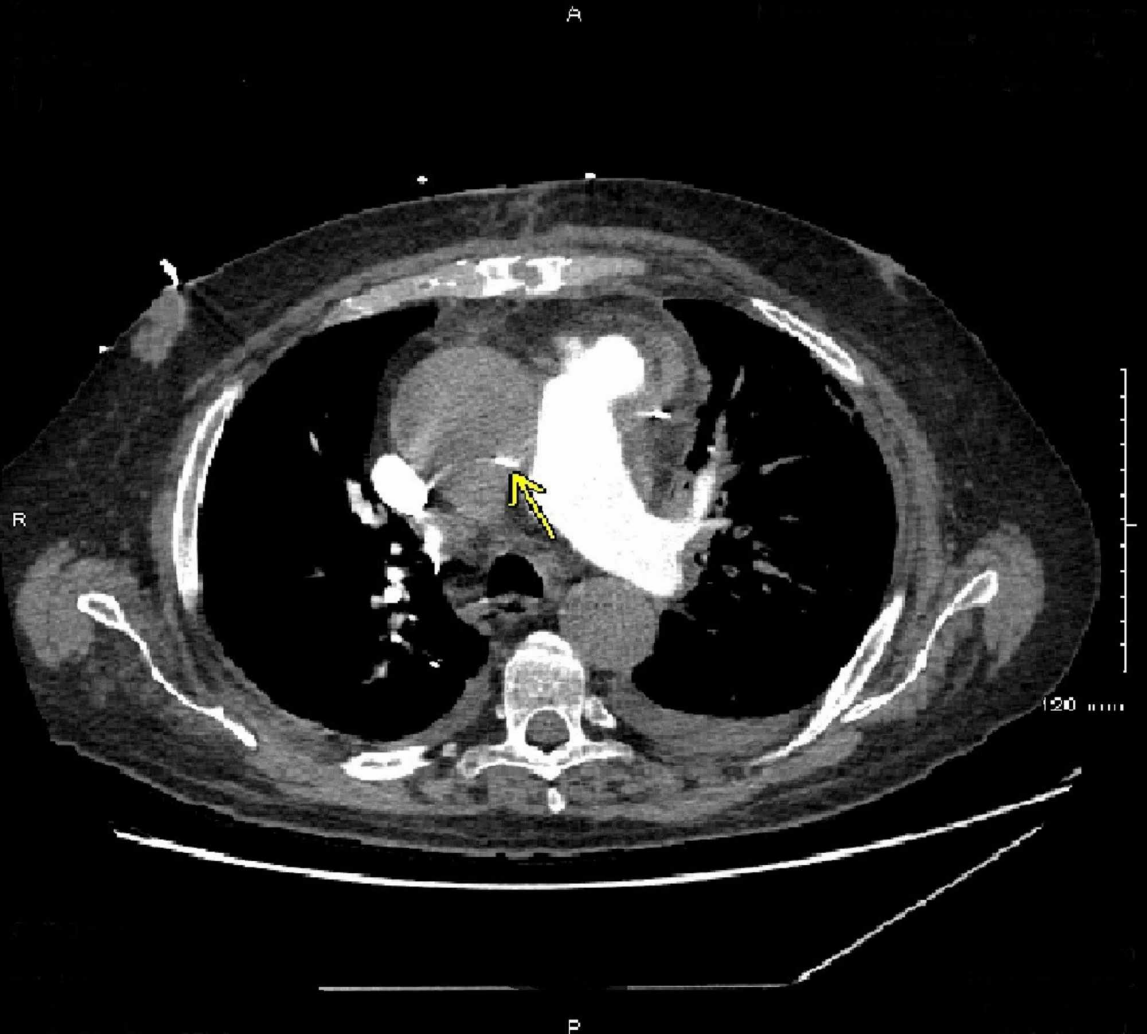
Computed tomography pulmonary angiogram (CTPA) concerning for aortic dissection CTPA shows a large ascending aortic aneurysm and displacement of intimal calcification (yellow arrow) concerning for Stanford type A aortic dissection.
